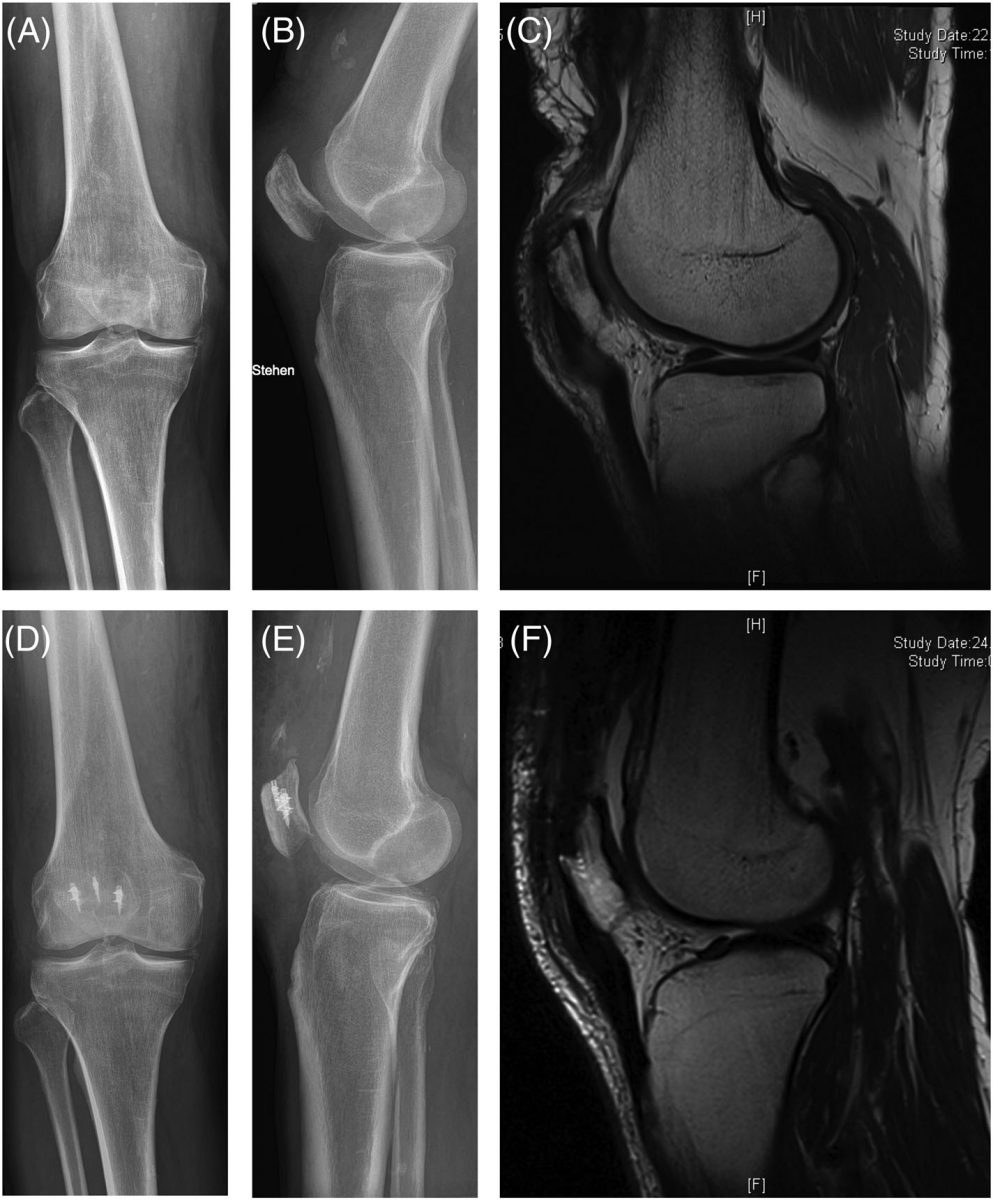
Radiographic images before and after quadriceps tendon reconstruction *(A)* Pre‐operative AP and *(B)* lateral X‐ray views of the right knee joint with a chronic quadriceps tendon rupture, visible in the *(C)* magnetic resonance imaging. *(D)* AP and *(E)* lateral X‐ray views of the reconstructed quadriceps tendon with titanium suture anchors placed in the patella. *(F)* The magnetic resonance imaging shows a reconstructed quadriceps tendon.
